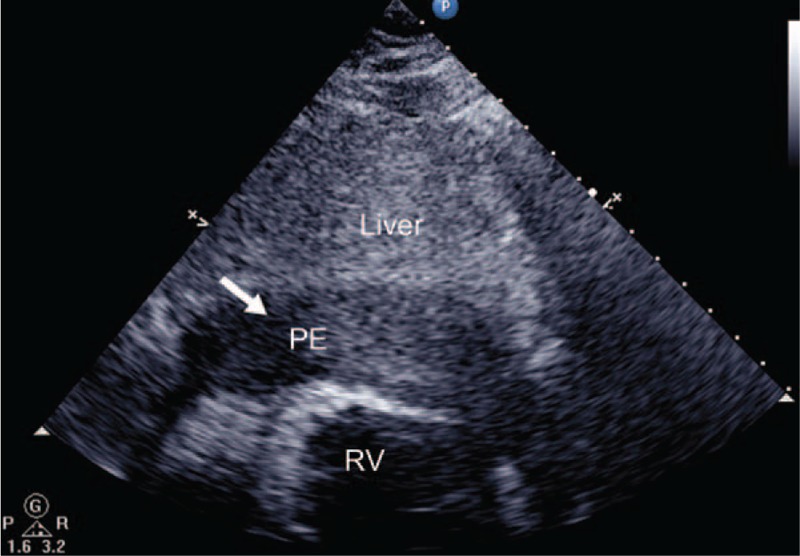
Echocardiography showing a massive hemorrhagic pericardial effusion (arrow).
